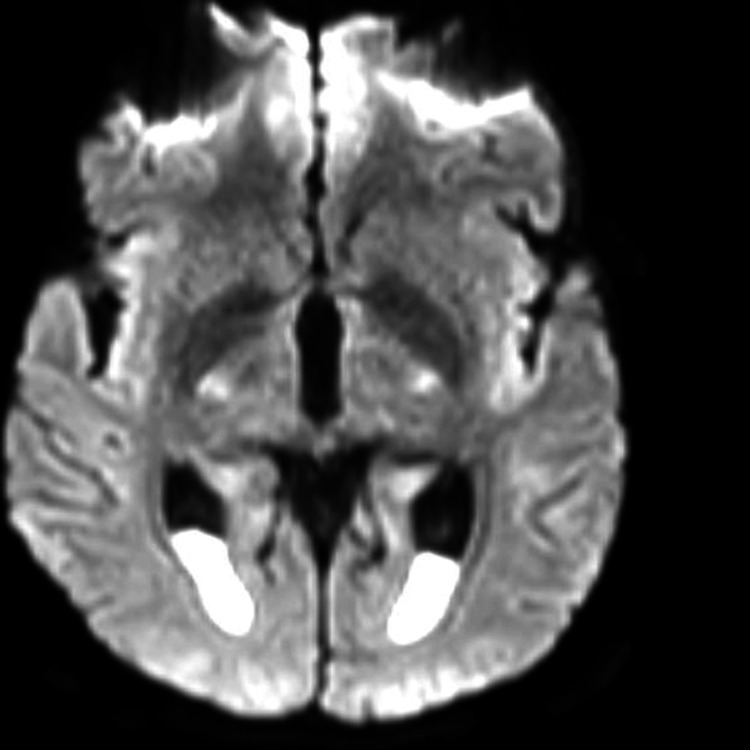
Axial DWI reveals hyperintense signal in the bilateral temporal and parieto-occipital lobes suggestive of restricted diffusion DWI: Diffusion weighted imaging
